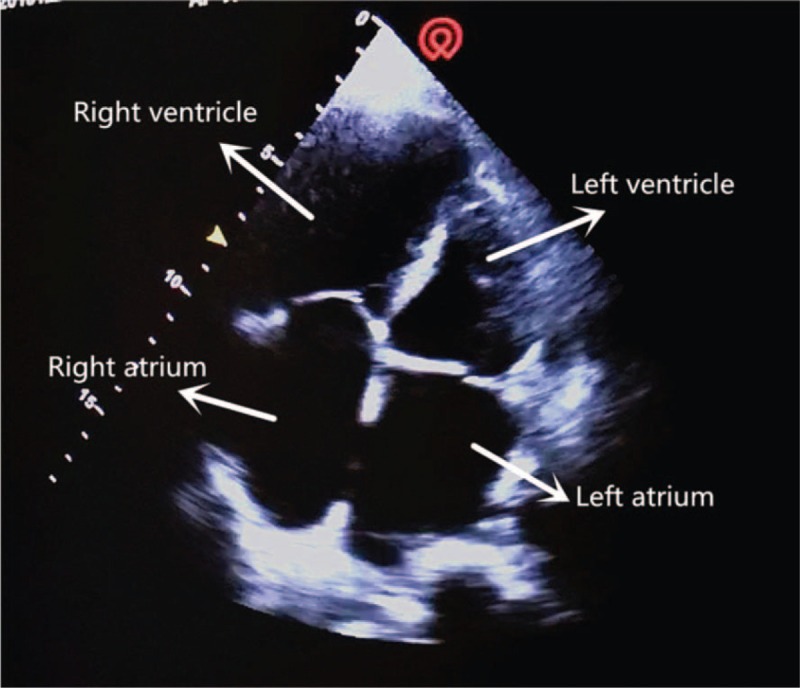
Apical 4 chamber section.
